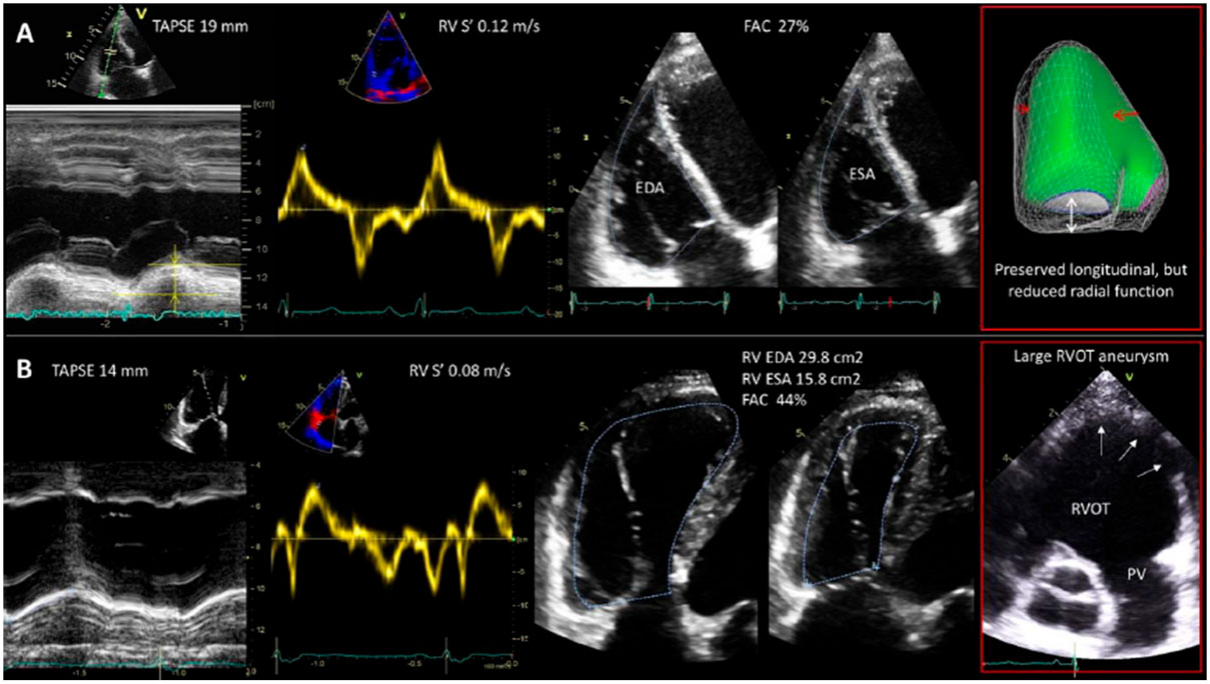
Importance of multi-parametric approach in accurate interpretation of RV systolic function by conventional echocardiography. (*A*) In a patient with pulmonary hypertension, TAPSE, and *S*′ values are normal, however FAC is reduced. 3D surface-rendered model of the RV demonstrates preserved longitudinal contraction (white arrow), but impaired radial contraction (red arrows) due to reduced RV-free wall motion and flattening of the interventricular septum. FAC considers not only longitudinal but also radial RV contraction and in this case is able to reflect the reduced RV systolic function. (*B*) In a patient with repaired Tetralogy of Fallot, TAPSE, and *S*′ are reduced (common post-cardiac surgery), but FAC is normal. 3D-derived RV EF is reduced (40%). This is explained by large dyskinetic area in the RVOT (last panel, arrows) which was not included neither in TAPSE nor in *S*′ or FAC measurements.
